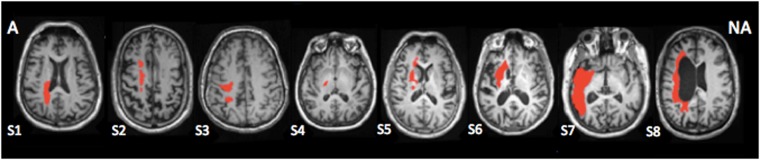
Lesion location. (A) Affected; NA: Non-affected. Location of the lesion superimposed in each individual T1-weighted image. The slice with the largest lesion size is presented.
